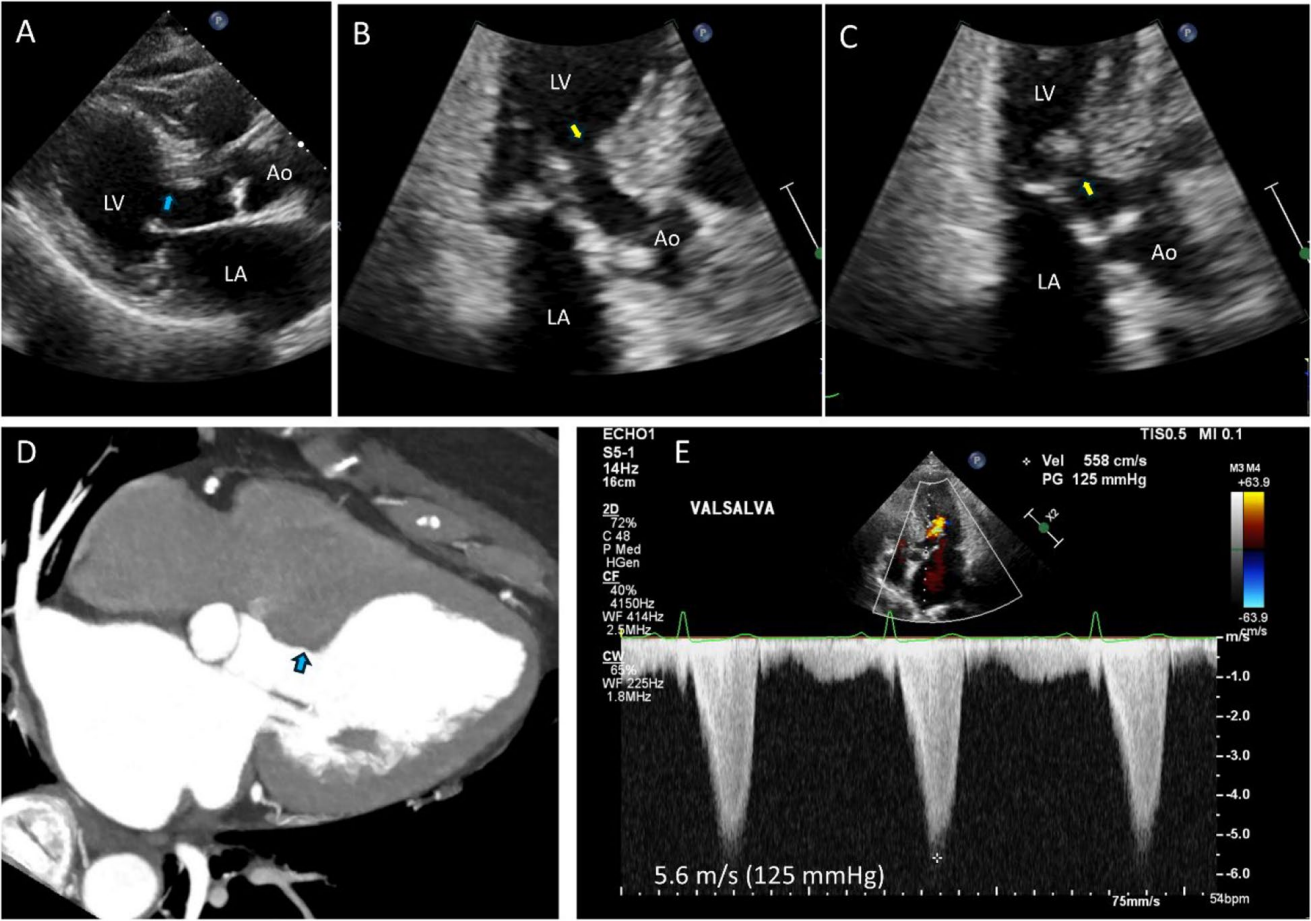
Representative echocardiographic and computed tomographic images of the sigmoid septum. (A, D) Parasternal long-axis view showing discrete septal thickening with characteristic angulation between the ventricular septum and aorta (blue arrows). (B, C) Prominent systolic anterior motion of the mitral valve contacts the septum, resulting in (E) elevated left ventricular outflow tract flow velocity and obstruction
